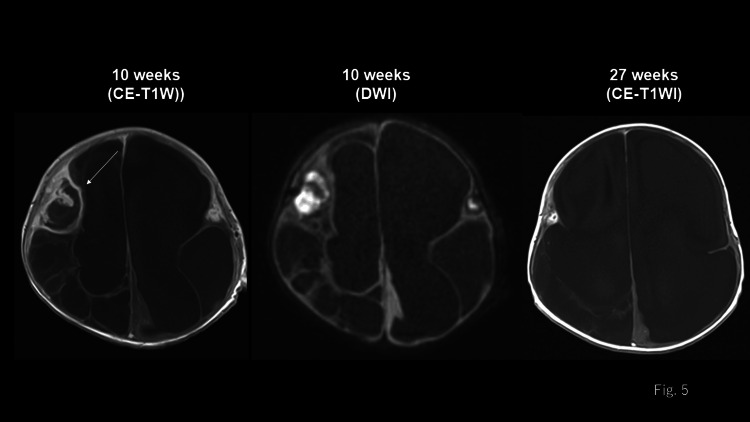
Serial contrast-enhanced-T1-weighted images of the brain Cystic parenchymal changes in the brain were noted on MRI 10 weeks after hospitalization. A diffusion-weighted image shows high signal intensity, suggestive of brain abscess bilaterally in the frontal lobes, and the contrast-enhanced T1-weighted images show ring enhancement (arrow). The MRI at 27 weeks revealed the progression of encephalomalacia. The brain abscess with ring enhancement is observed to be reduced in size.
